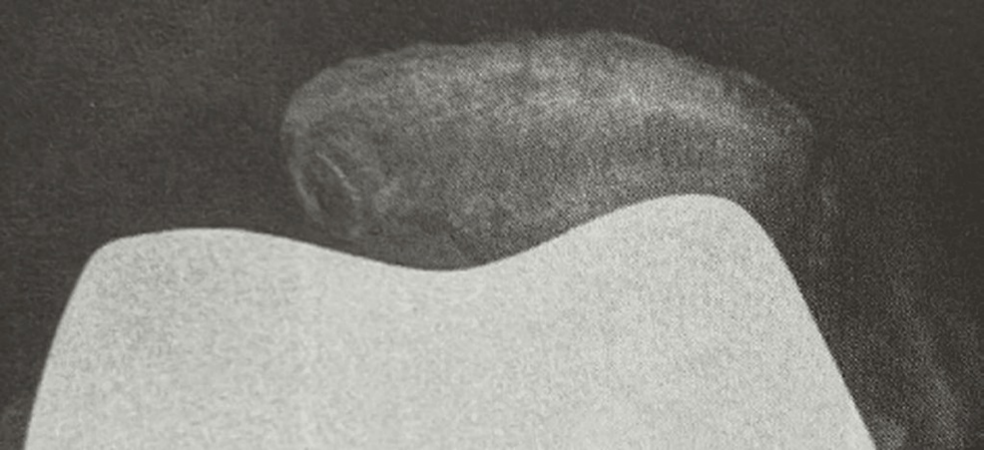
Postoperative skyline radiograph of a 62-year-old male suffering from hyper-compression syndrome of the patella leading to anterior knee pain
